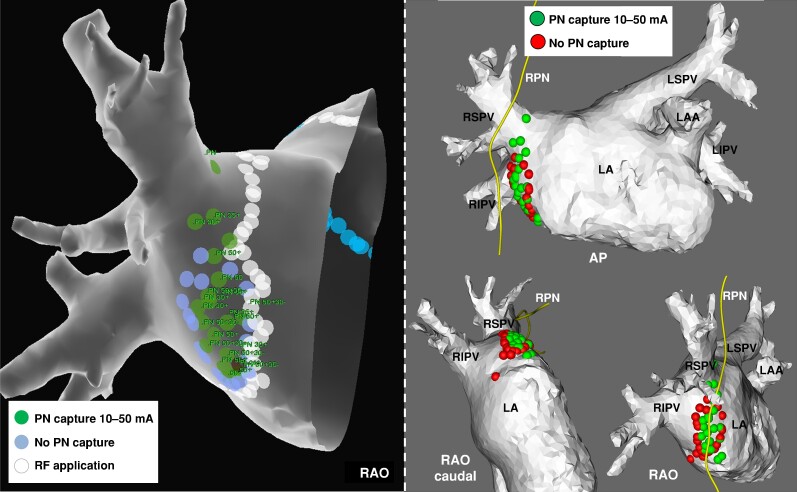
Left panel: electroanatomical mapping of the septal portion of the right pulmonary veins ostium, showing RPN pace-mapping sites labelled with the capture threshold. Right panel: 3D reconstruction of the CT scan, with merging of the pace-mapping sites, using MATLAB software. The RPN is segmented in yellow. For facilitating visualization of capture and non-capture sites, all capture sites are displayed in green, regardless of the threshold. LA, left atrium; LAA, left atrial appendage; LIPV, left inferior pulmonary vein; LSPV, left superior pulmonary vein; RA, right atrium; RIPV, right inferior pulmonary vein; RPN, right phrenic nerve; RSPV, right superior pulmonary vein; SVC, superior vena cava.
